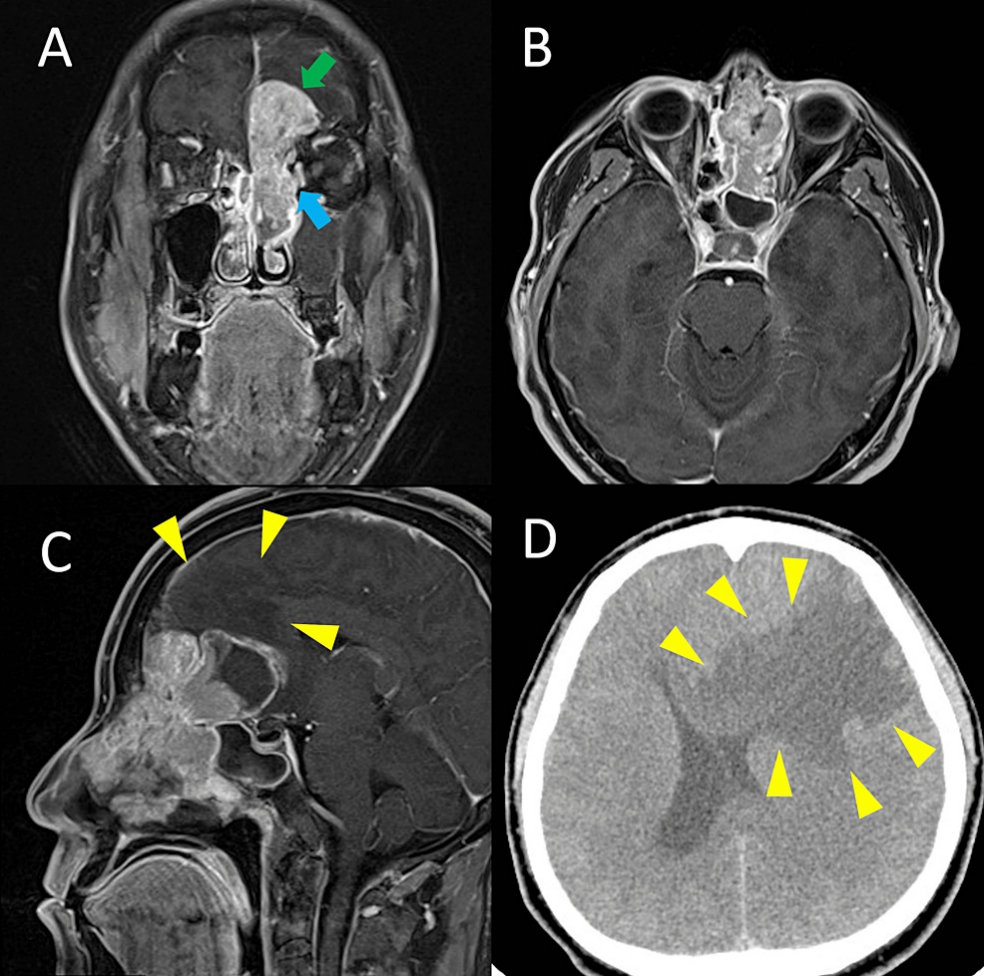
Imaging findings at initial examination and seven days after the initial examination Contrast-enhanced MRI shows the large tumor occupying the left nasal cavity with extension into the ethmoid sinus, frontal sinus, and sphenoid sinus [coronal(A), axial (B) and sagittal (C) plane]. The tumor extends to the frontal lobe (A: green arrow), exerting local pressure on the medial orbital wall (A: blue arrow) and infiltrating the anterior skull base. There is large perifocal edema in the frontal lobe (C: yellow arrowhead). (D) CT image seven days after the initial examination shows further progression of the brain edema (D: yellow arrowhead).
